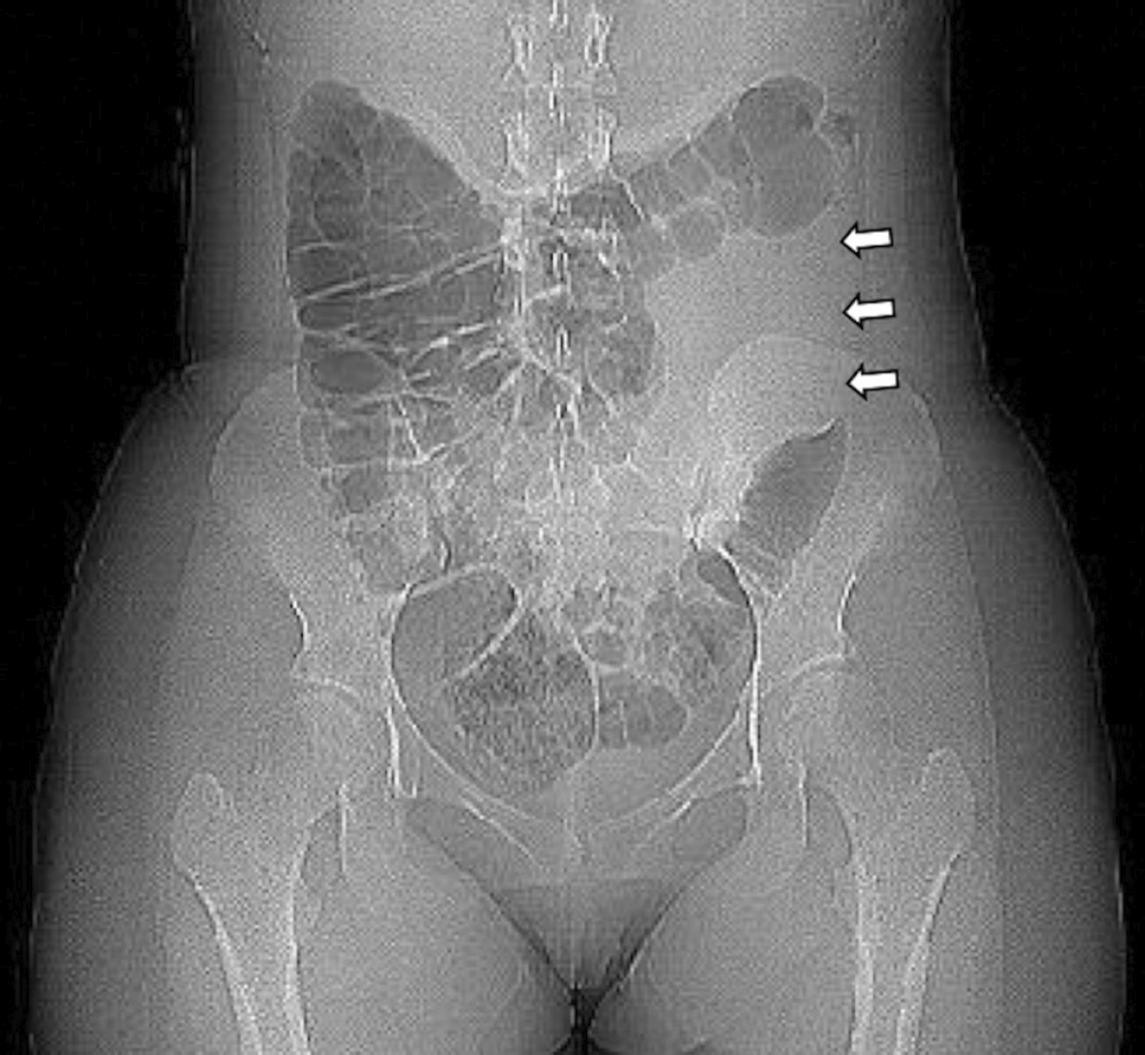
CT scout image. Intestinal gas is interrupted from the splenic flexure to the middle of the descending colon (arrow).
